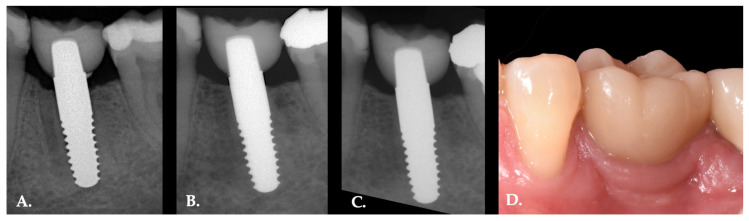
Intraoral radiographs of a single piece zirconia implant placed in 36 in a 39-year-old female patient at baseline (A), 4 years (B) and 11 years (C) after implant placement. Intraoral photograph of the definitive restauration 11 years (D) after implantation.
